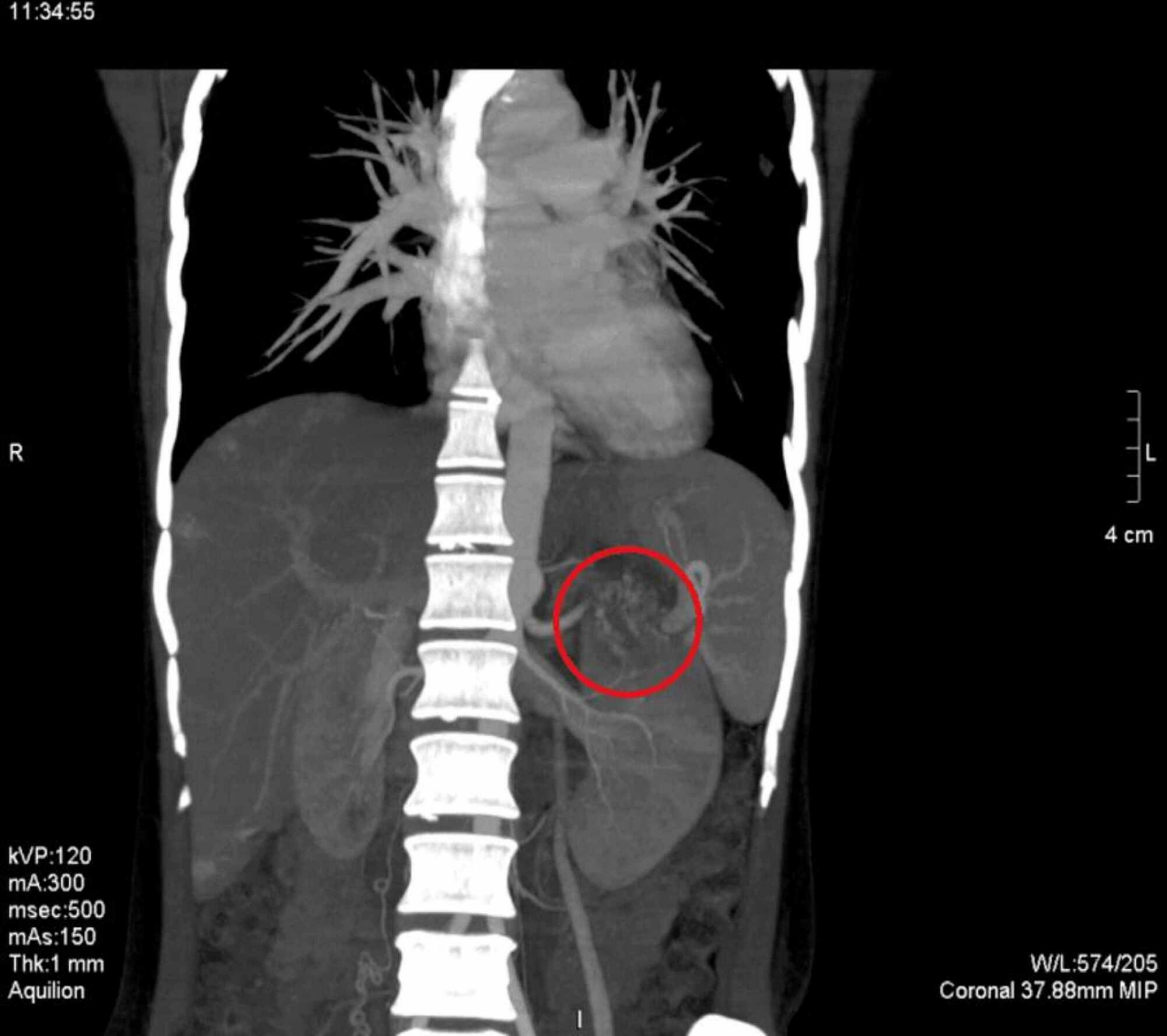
CT angiography shows the renal lesion to be hypervascular and fed by the branches from the aorta CT: computed tomography
